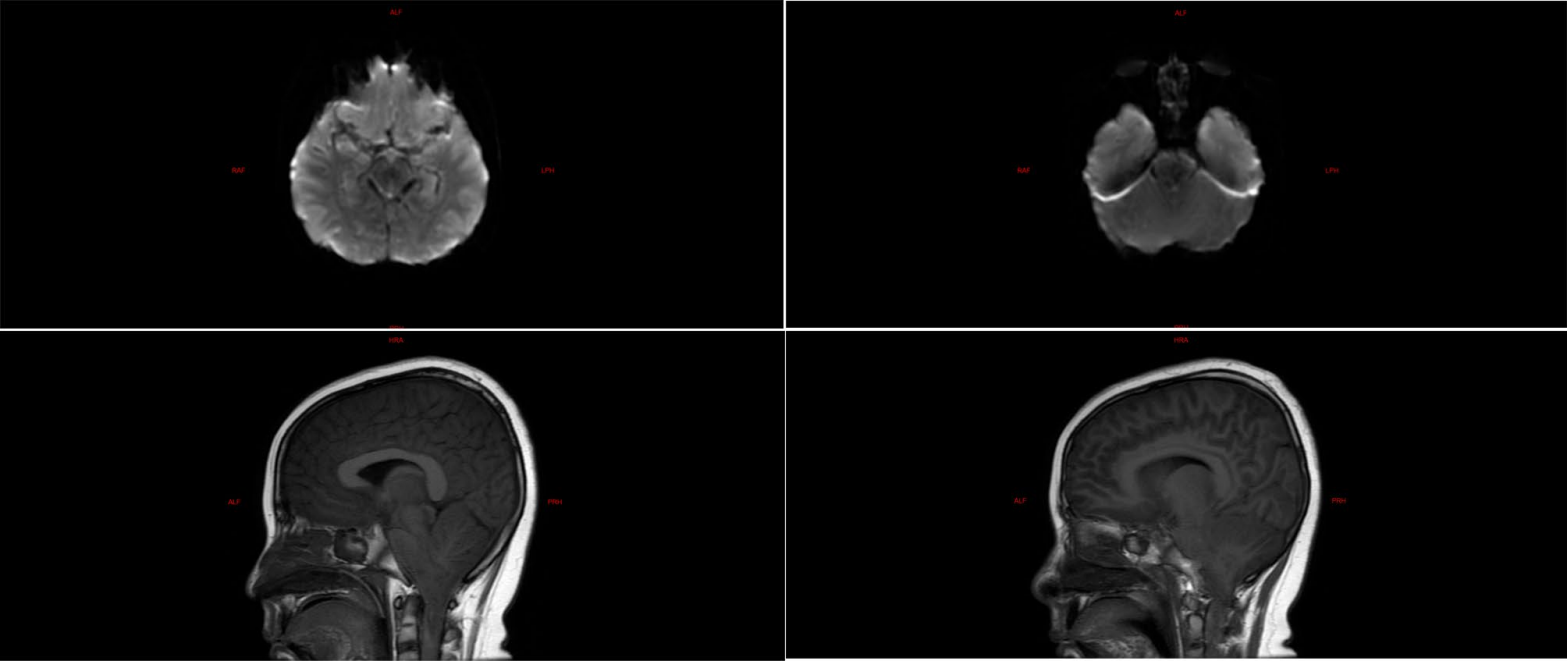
Brain MR images of case 3. a, b are DWI sequences, showing bilateral temporal and occipital lobes symmetrical diffuse high signal; c, d are T1 WI sagittal sequences, showing cerebellar sub-tonsillar herniation. The patient developed herniation of the brain induced by fulminant cerebral edema, exhibiting rapidly progressing decerebrate rigidity shortly after admission, accompanied by central diabetes insipidus. Unfortunately, the patient passed away after the decision to discontinue treatment
